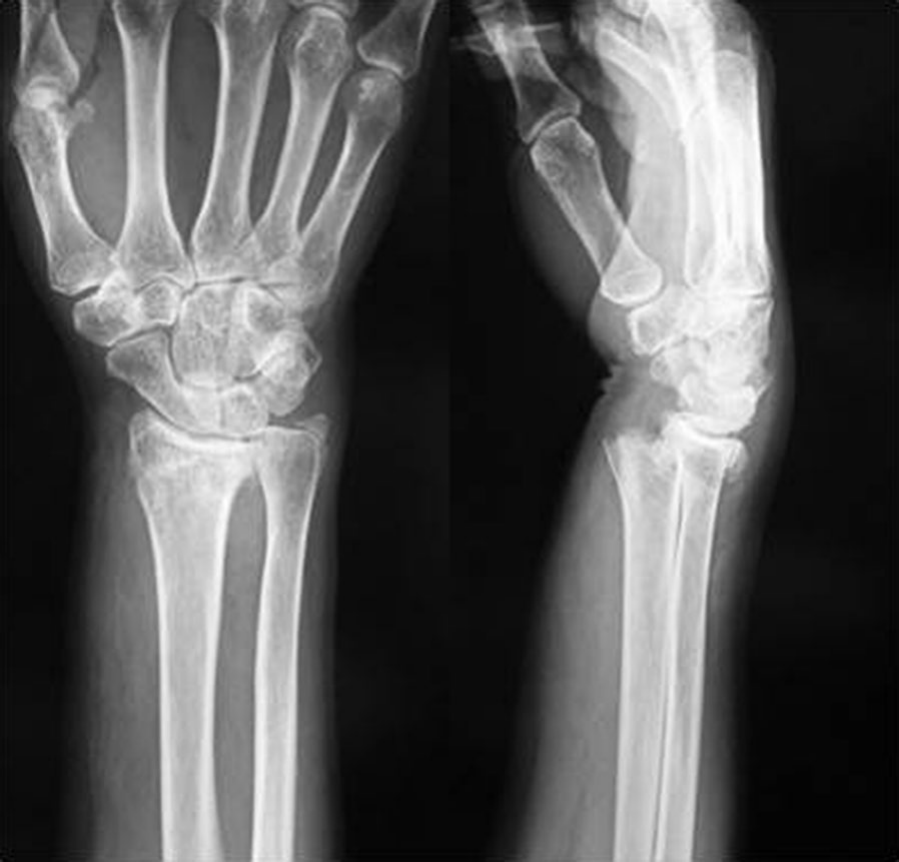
X-rays of the right hand at the first examination showing the distal radius fracture with a rim fragment
